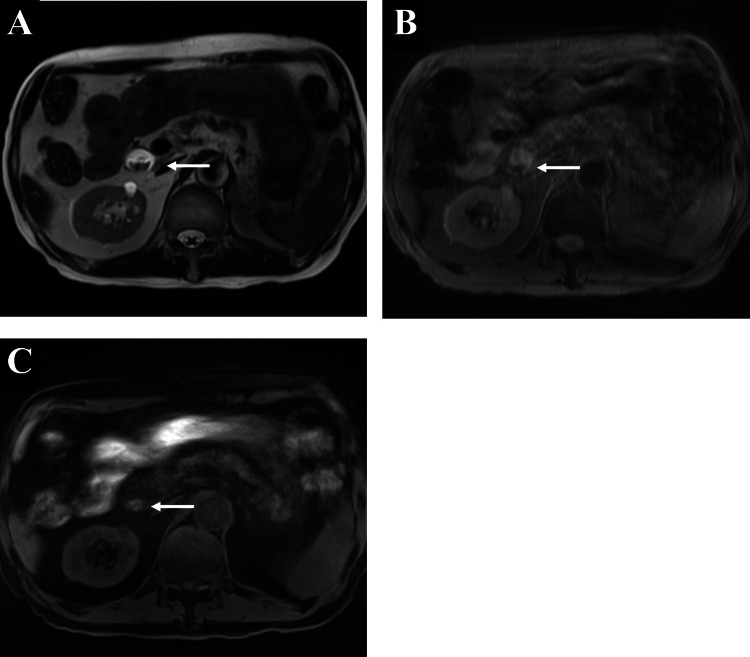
Magnetic resonance imaging after the recurrence of epigastric pain. The lesion in the extrahepatic bile duct (arrow) had low signal intensity on (A) T2- and (B) T2 star-weighted images and intermediate to high signal intensity on (C) T1-weighted images.
